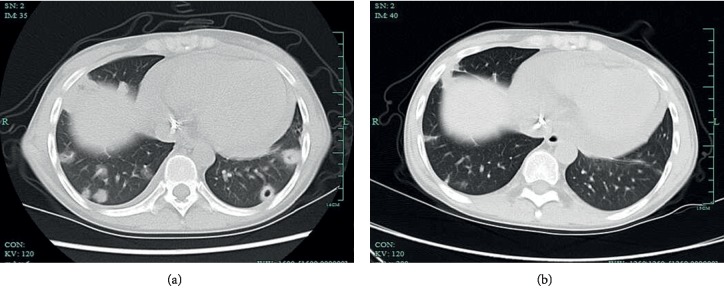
A 20-year-old woman with a history of KT 4 years ago. (a) Chest CT in the lung window shows subpleural multiple nodules, some with cavitations, located in bilateral lungs. (b) Follow-up chest CT (2 months after antifungal treatment) shows the resolution of the pulmonary lesions.
